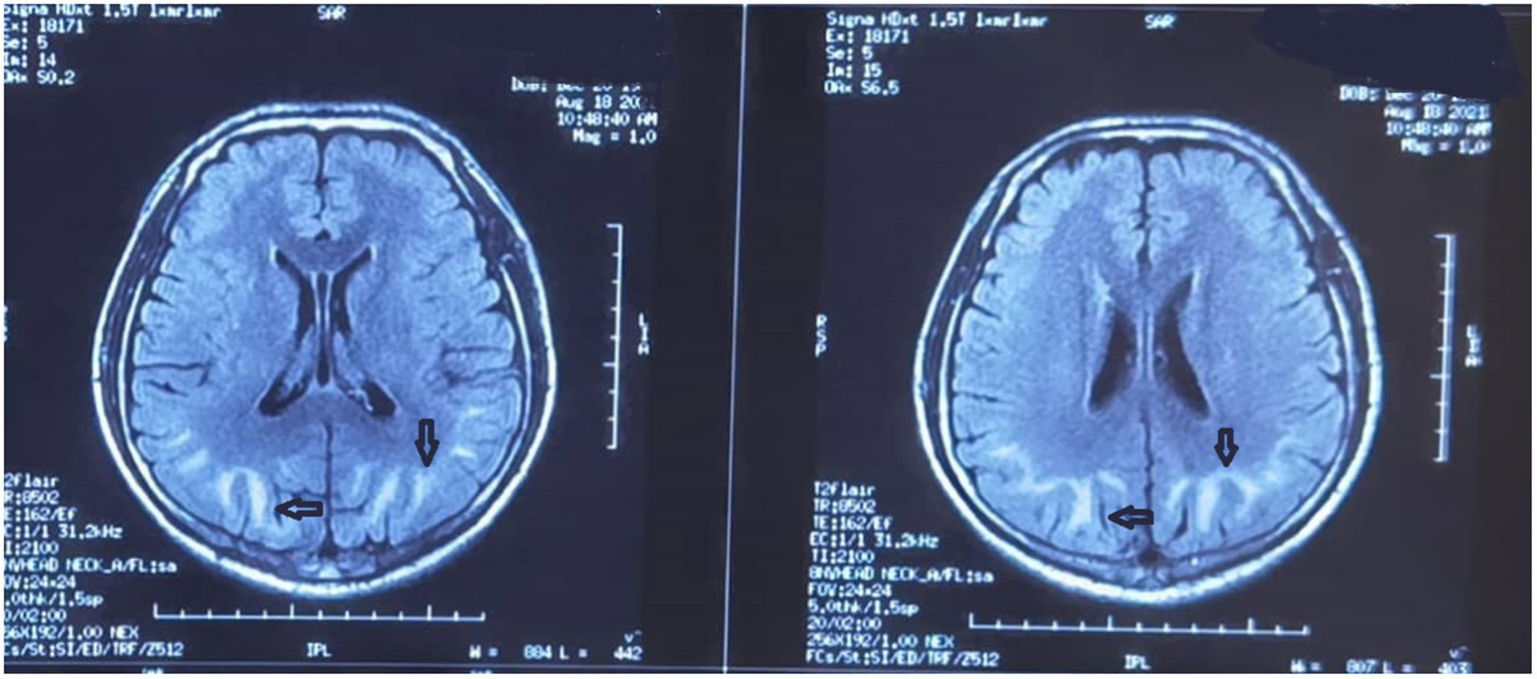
Brain magnetic resonance image obtained on the 3rd day after admission. Strip-like long T1 and T2 signal shadows in the subcortical white matter of both cerebral hemispheres are evident. There is a high intensity on both T2-fluid-attenuated inversion recovery and diffusion-weighted imaging. A fuzzy demarcation is visible between the skin and medulla. The septum pellucidum is widened and the midline structures are centered.
